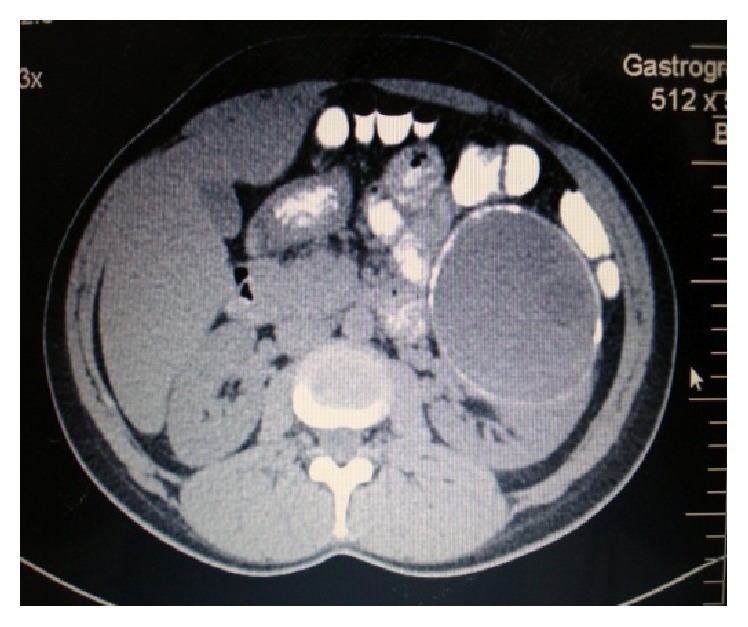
CT imaging revealing the 14 cm left renal cystic mass.
